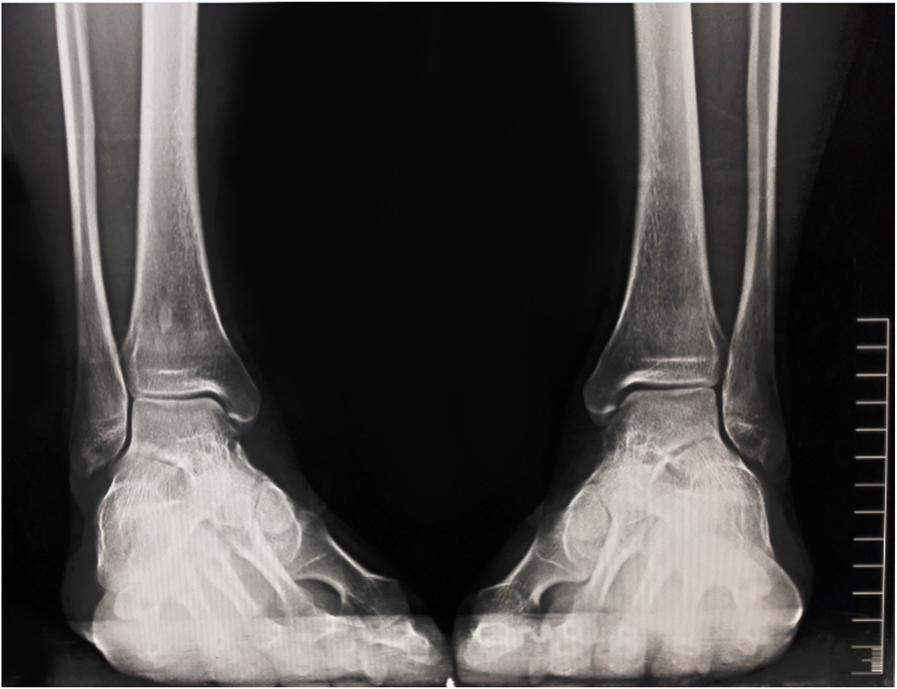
Ankle mortise view x-ray photography. The lucent area of tibia-talus or fibula-talus joint is about 4 mm, including a small amount of synovial fluid and 2 mm + 2 mm non-visualized articular cartilage
